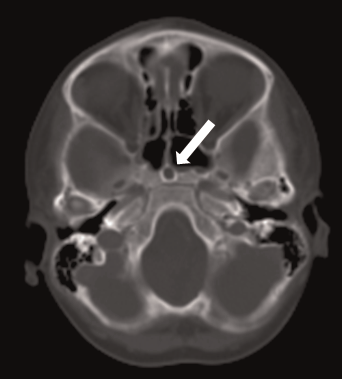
Axial CT scan of the head in bone window showing a round corticated defect (arrow) in the sphenoid body.
